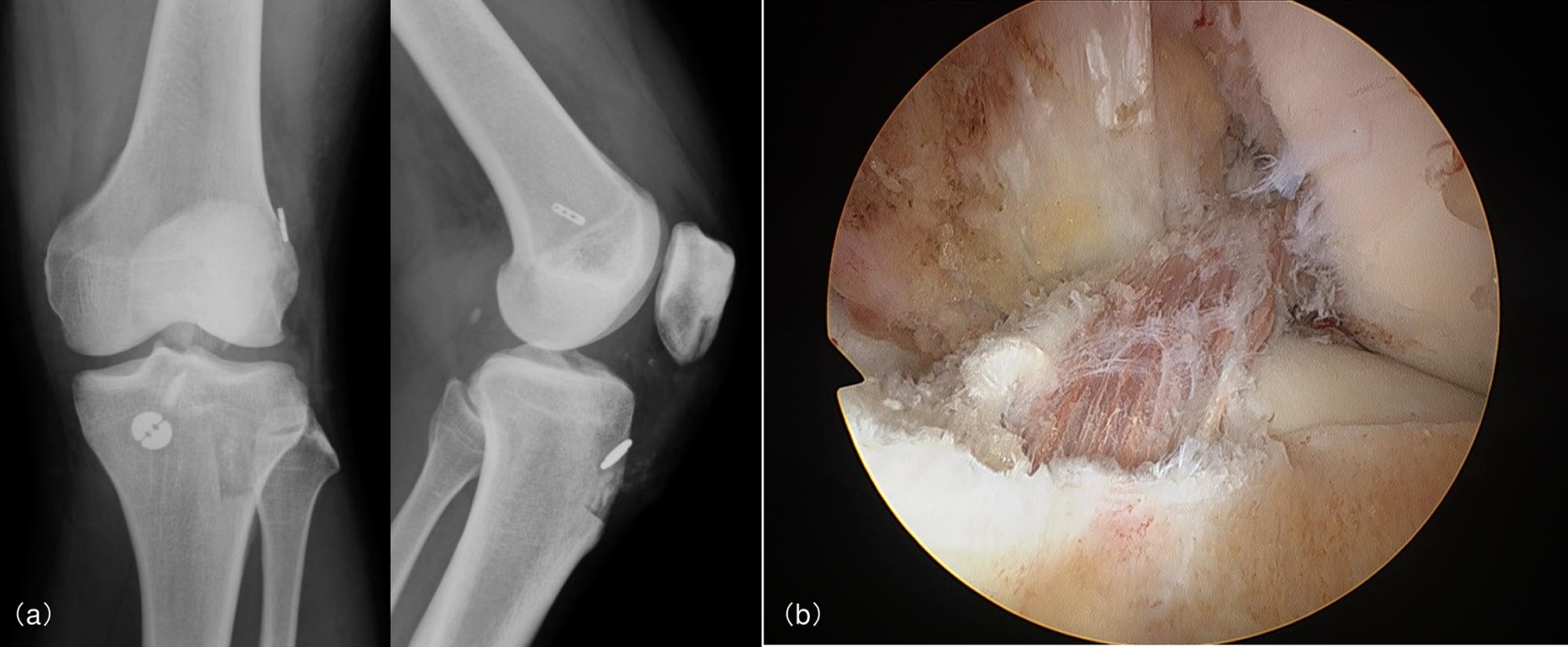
Post-operative radiographs and arthroscopic view of the left knee after surgery. (a): Post-operative radiographs ((fixation to the femur by BTB TightRope (Arthrex, Naples, FL) and to the tibia by ABS button (Arthrex, Naples, FL)) (b): Arthroscopic view.
